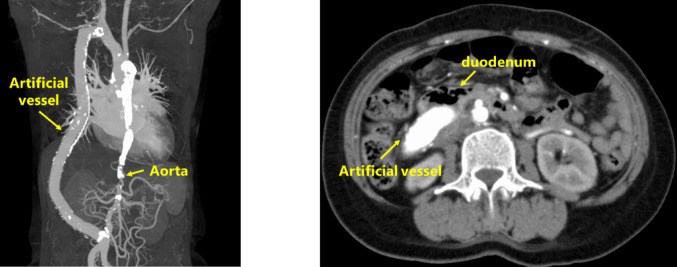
Computed tomography (CT) findings. CT images showing the artificial blood vessel positioned near the duodenum and compressing the descending duodenum, with no obvious active bleeding. CT, computed tomography
